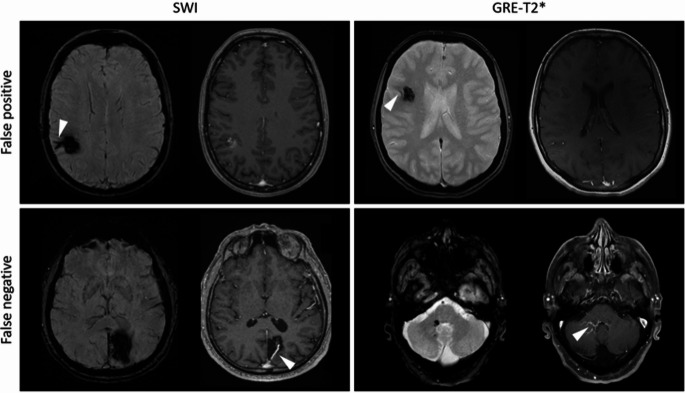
Exemplary cases of misdiagnosis. Axial MRI images are shown. SWI or GRE-T2* sequences are shown, next to the CE-T1 sequences of the same slice. The existing or suspected DVA is highlighted with an arrow
